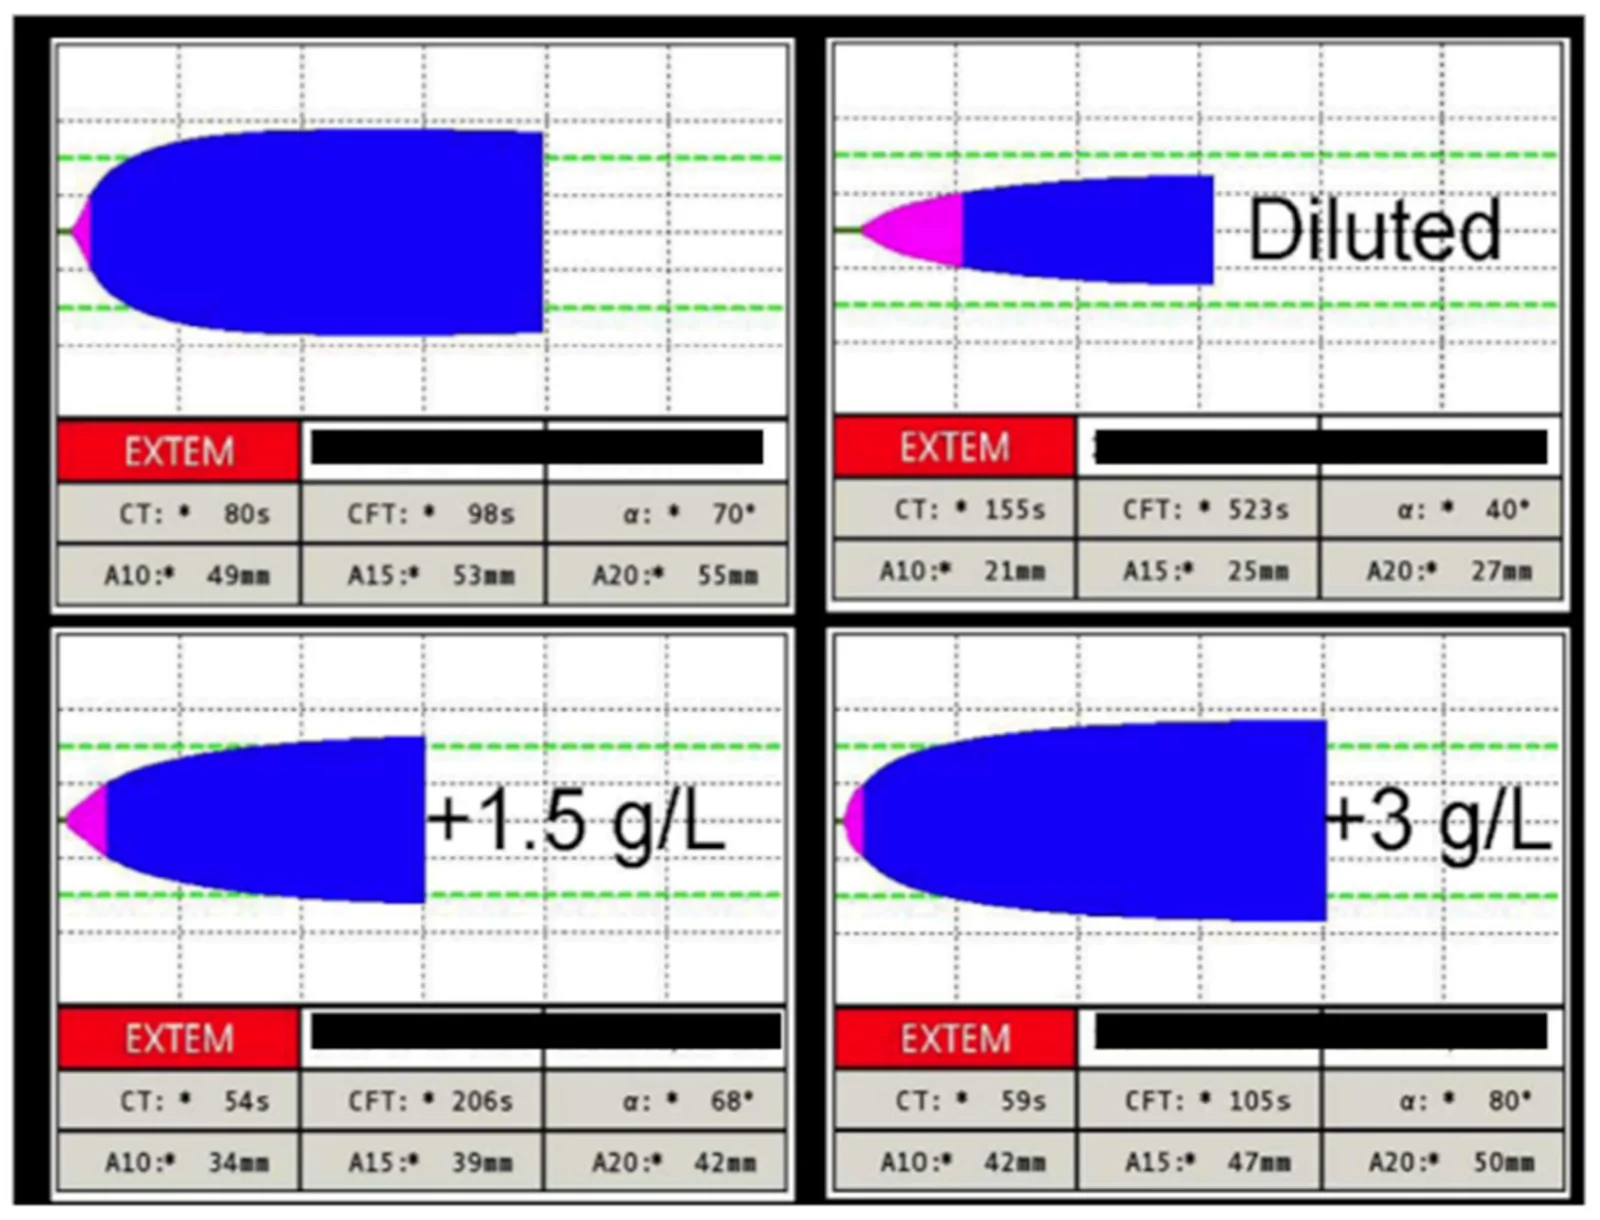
Addition of fibrinogen to diluted whole blood in vitro restores rotational thromboelastometry (ROTEM) parameters. These representative thromboelastometric traces represent how addition of fibrinogen in EXTEM (ROTEM with clotting initiated by tissue factor) improved all thromboelastographic parameters (clotting time (CT), the lag time before clot formation, clot formation time (CFT), α, the angle which reflects the initial rate of clot formation, and A10, firmness amplitude at 10 min (Reprinted with permission from Bolliger et al. 2009; Br J Anaesth 102(6):793–799 [142] with permission from Elsevier).
